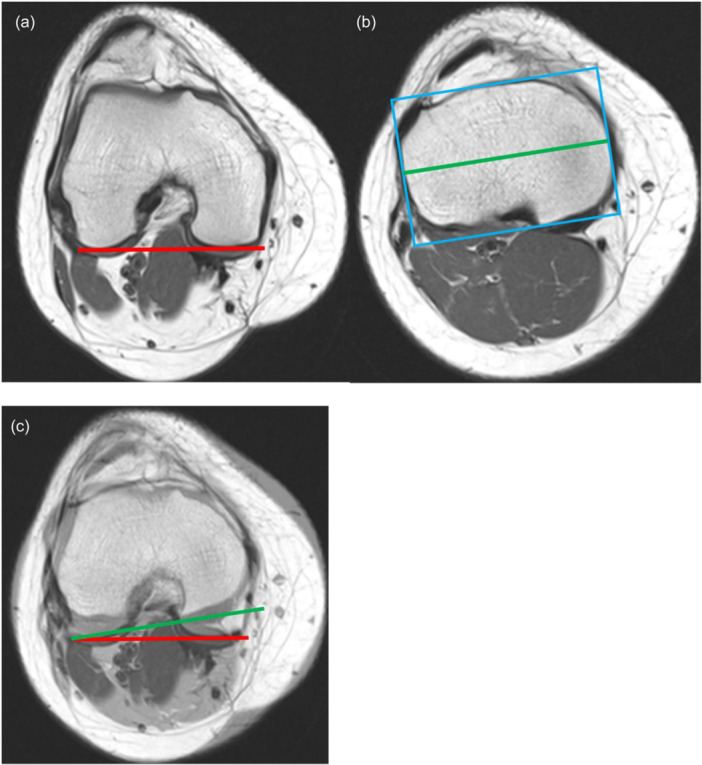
Plateau‐anchored MRI TFR method, right knee (same patient as Figures 1 and 2). (a) Femoral posterior condylar line at the slice of maximal condylar AP diameter. (b) On the first tibial slice below the menisci at the PCL tibial insertion, a minimum‐area bounding rectangle is placed snugly around the tibial plateau without subsequent manual rotation; the tibial ML axis is the line connecting the midpoints of the rectangle's short sides. (c) Superimposition of femoral and tibial lines; TFR is the acute angle between them (positive for tibial external rotation). Example: 10°. AP, anteroposterior; ML, medial–lateral; MRI, magnetic resonance imaging; PCL, posterior cruciate ligament; TFR, tibiofemoral rotation.
